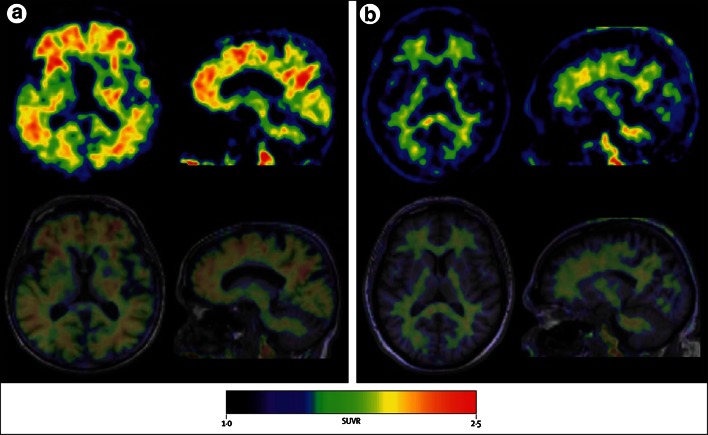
^18^F-florbetaben PET detects Alzheimer’s disease with high sensitivity and specificity. PET images (*upper row*) and PET/MRI overlay (*lower row*) of two paradigmatic cases: a a patient with Alzheimer’s disease dementia, b a healthy control individual, imaged within a worldwide multicenter trial. While in the healthy control, only unspecific uptake in the white matter was observed, this extended to the outer gray matter in the Alzheimer’s disease dementia patient. Reprinted from ref. [26], Copyright (2011) with permission from Elsevier
